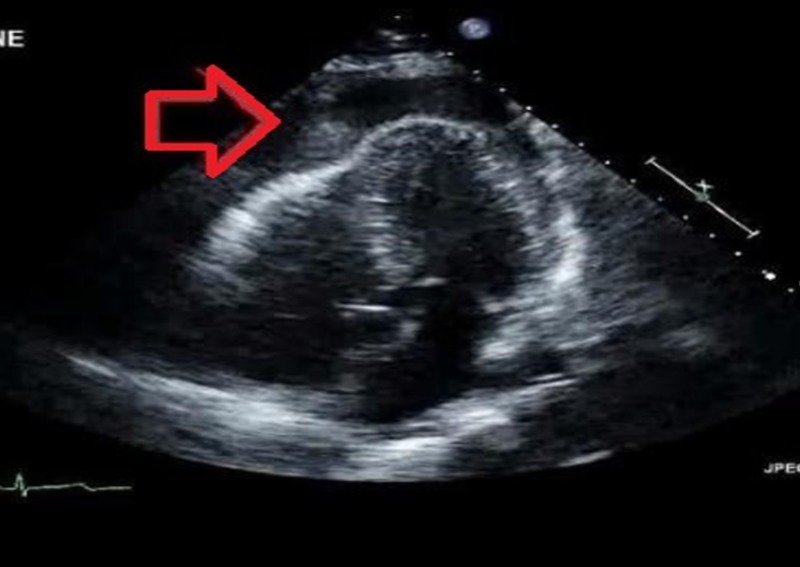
Transthoracic echocardiogram showing circumferential pericardial effusion
